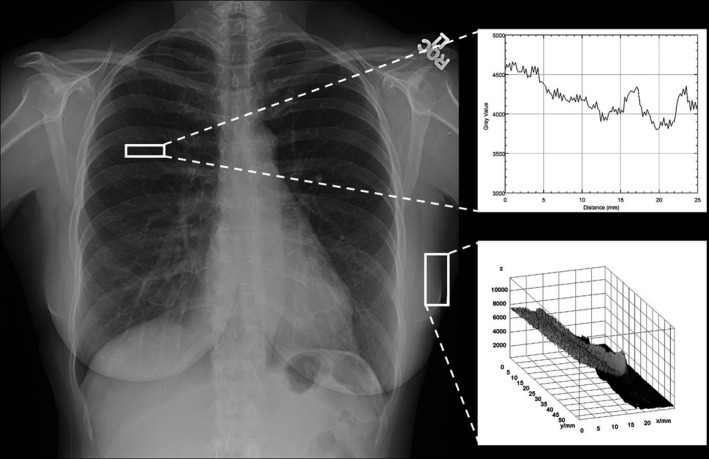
Corrective action was successful. PA chest radiograph of first female patient taken after detector recalibration showed less prominent grid lines and dramatically reduced rebound artifacts. Upper right inset: line profile shows grid lines are less pronounced. Lower right inset: surface plot demonstrates reduced rebound artifact.
